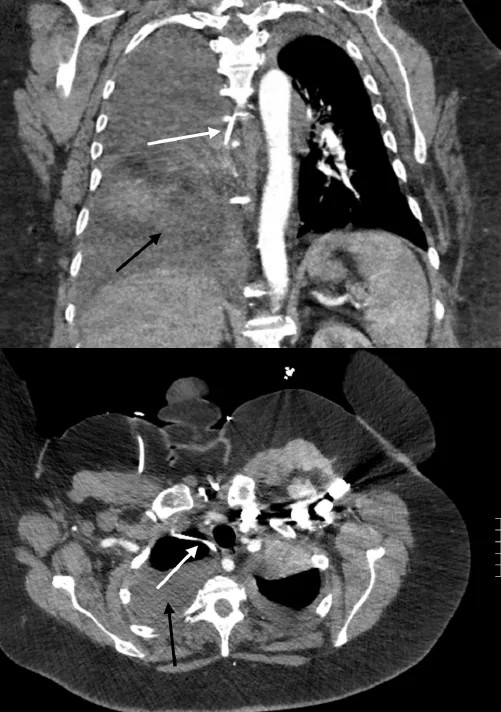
Sagittal (top) and axial (bottom) views from a contrast-enhanced chest computed tomography chest demonstrating a large right pleural effusion (black arrows) adjacent to a central venous catheter (white arrows) of uncertain location.
